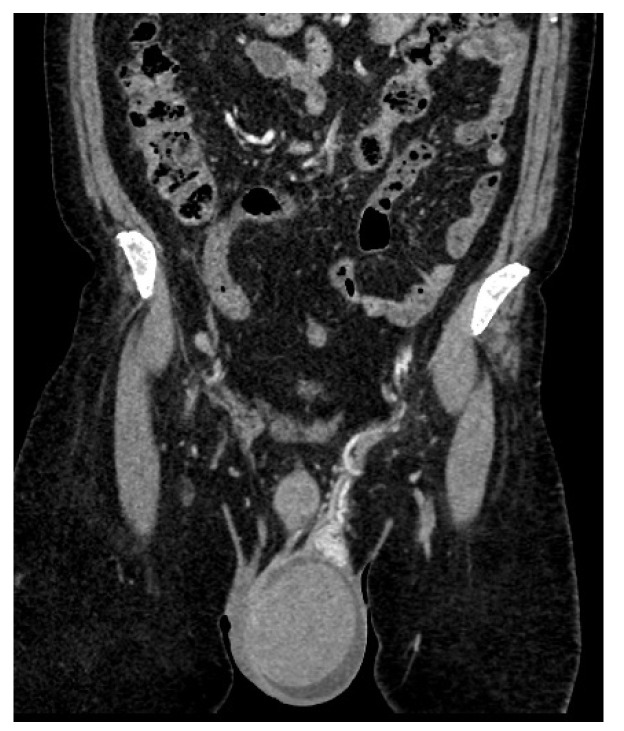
Abdomino-pelvic CT with contrast substance, coronal plane sections: Left testicle.
